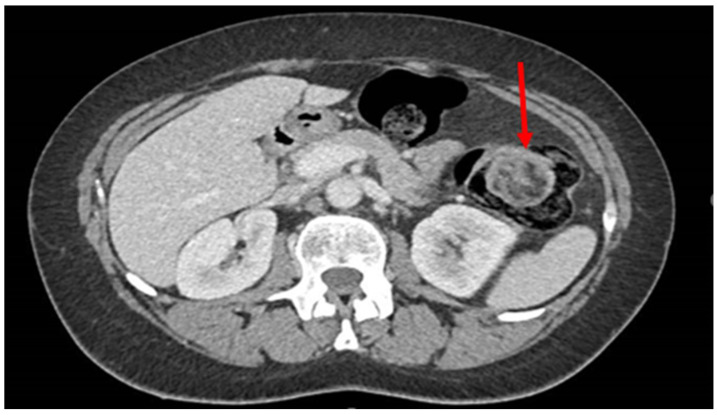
CT scan image of a colon lipoma. CT scan image of a colon lipoma (red arrow).
